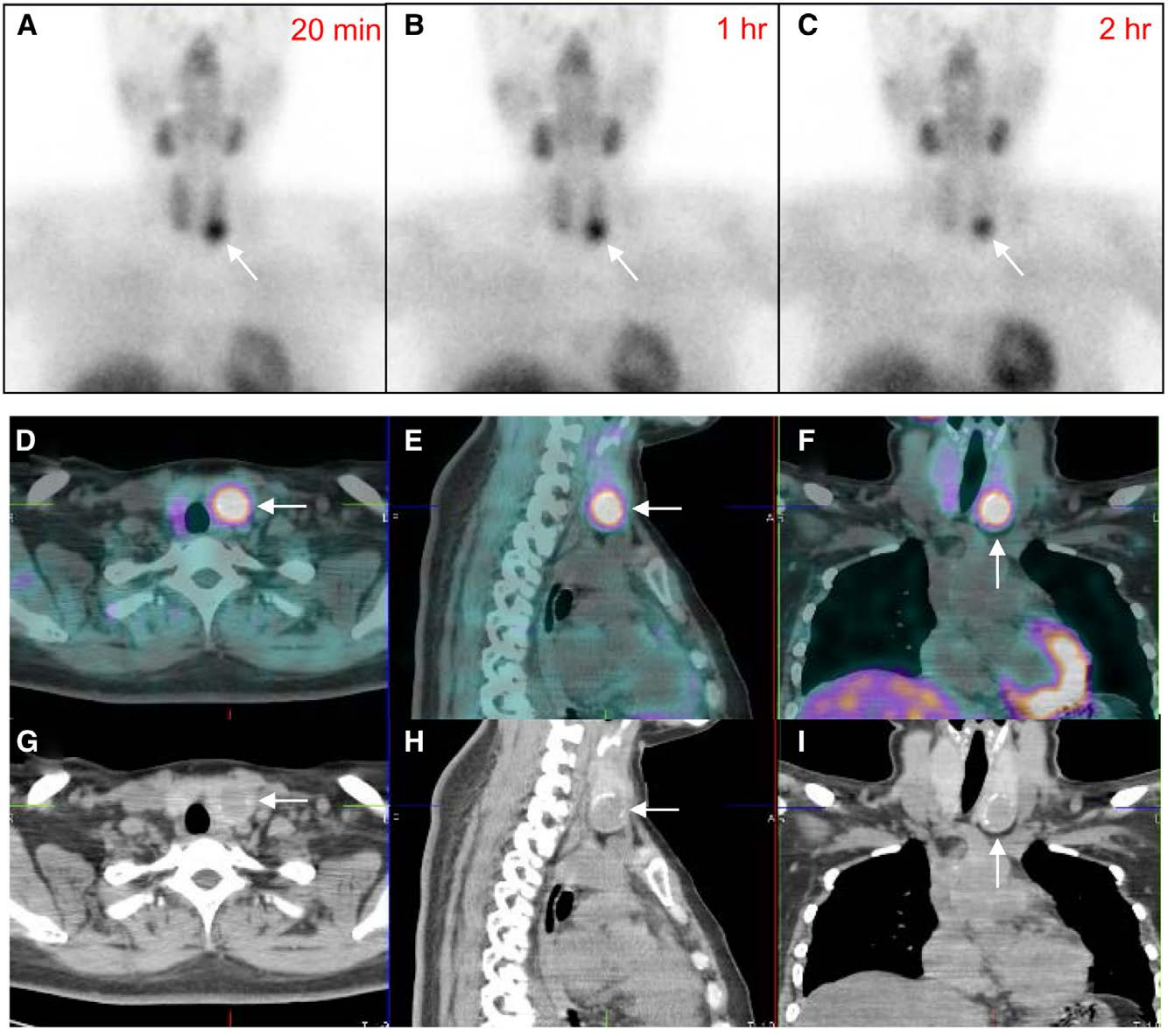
Initial Tc-99m MIBI scan and SPECT/CT. The 20-min image showed focally increased uptake in the left thyroid gland area (A). The uptake continued on the 1-h and 2-h images (B, C). The SPECT/CT revealed a 2.1-cm nodular lesion within the left thyroid gland exhibiting high Tc-99m MIBI uptake (D–F, fusion images, G–I CT images). Arrows indicate the lesion.
